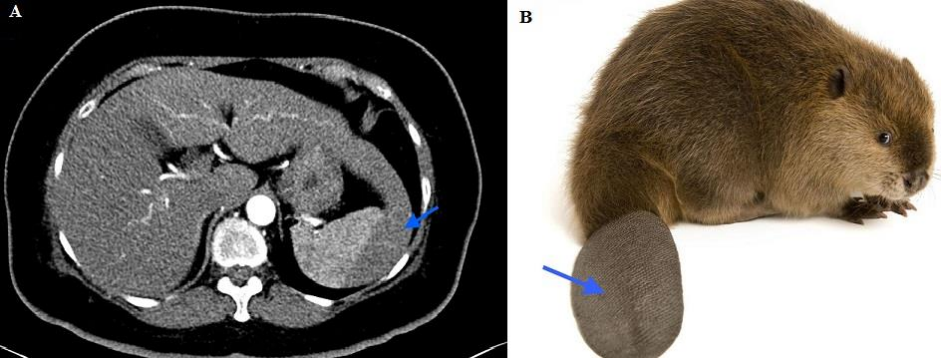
(A) abdominal CT (Computed tomography) in axial section, showing the ‘beaver tail liver’ (arrow) otherwise known as an elongated left lobe of the liver; this is seen extending beyond the spleen, almost encasing it entirely; (B) note the resemblance of the tail of a beaver (arrow) to the appearance of the elongated left liver lobe (A)
